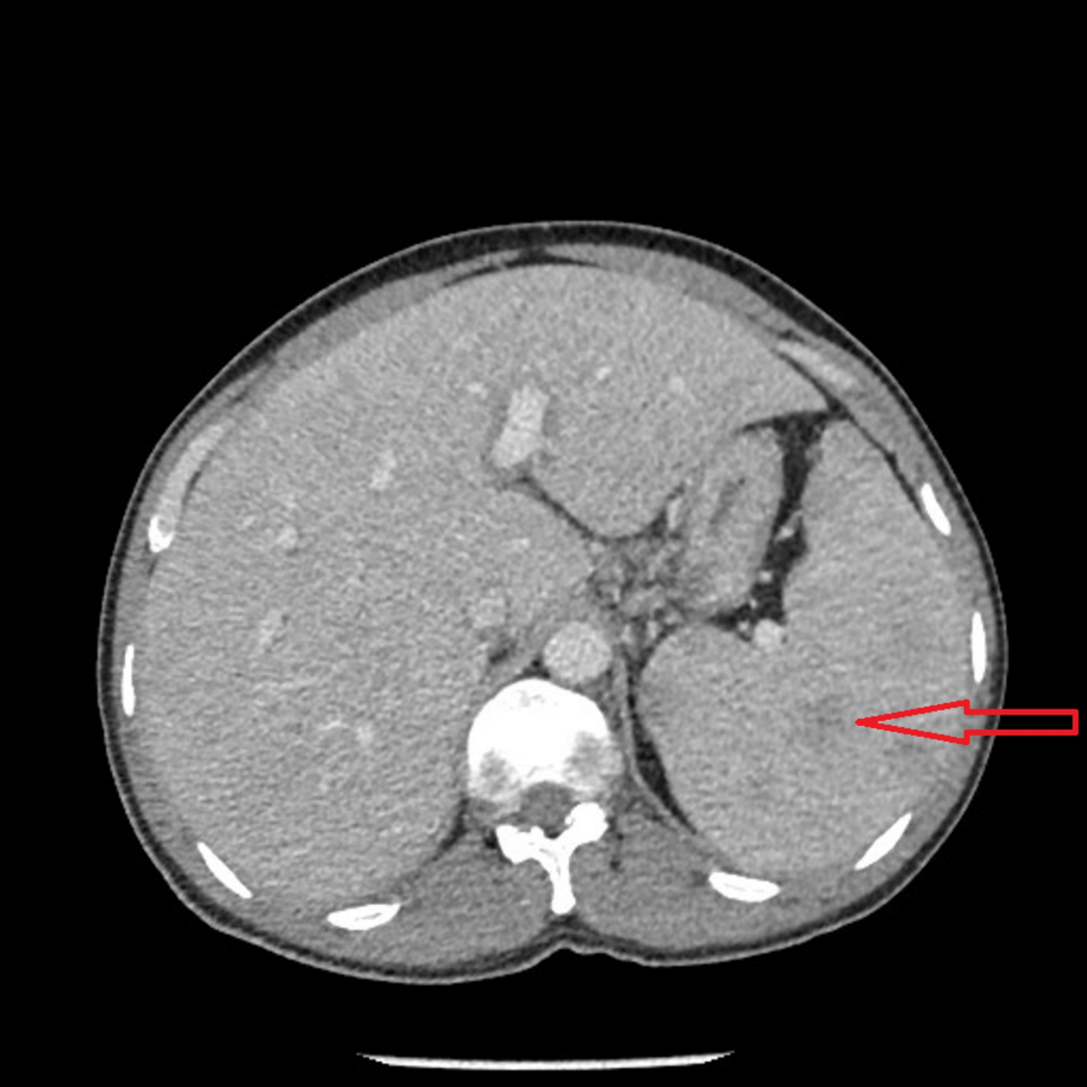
CT abdomen with contrast. CT abdomen with contrast reveals moderate hepatosplenomegaly with the red arrow pointing towards hypodense lesions in the spleen. CT: Computed tomography
